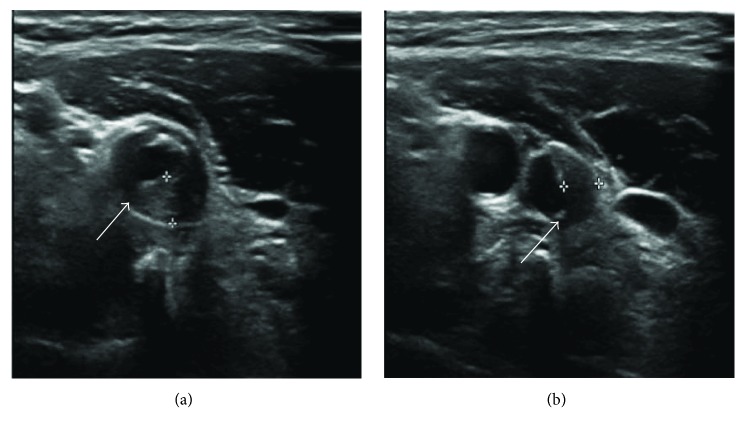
Carotid artery color Doppler imaging showed carotid artery plaques and stenosis. (a) The stenosis of the left common carotid artery is 80% (arrow). (b) The stenosis of left internal carotid artery is 60–70% (arrow).
